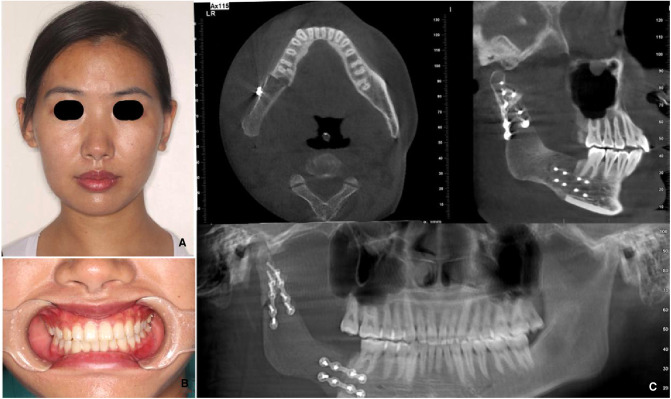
Postoperative anterior view showing that the mandible is symmetrical for 3 months after operation (A); postoperative CBCT demonstrating that both the shape of the mandible and the height and width of the alveolar crest are satisfactory (B). L stands for the left side, R stands for the right side, both sides are ruler.
